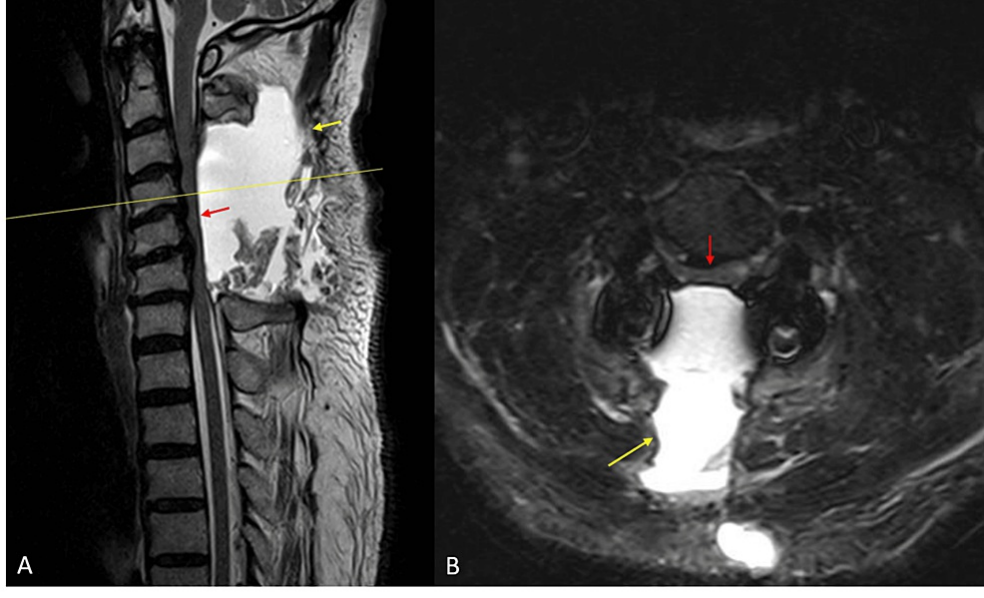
Sagittal (A) and axial (B) MRI on postoperative day eight demonstrating a large fluid collection (yellow arrows) compressing the spinal cord (red arrows)
